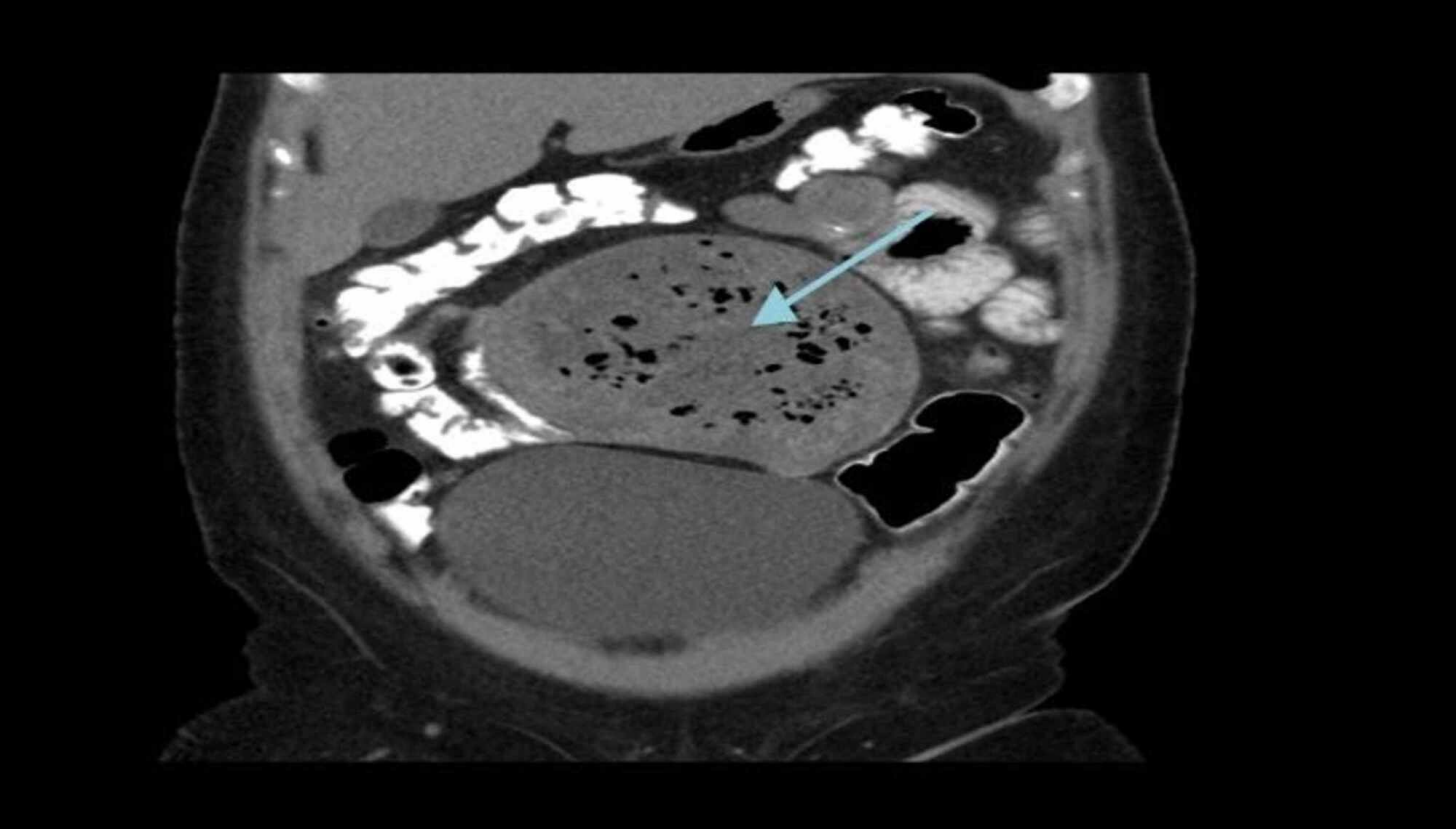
CT Abdomen showing huge intra-abdominal mass.
